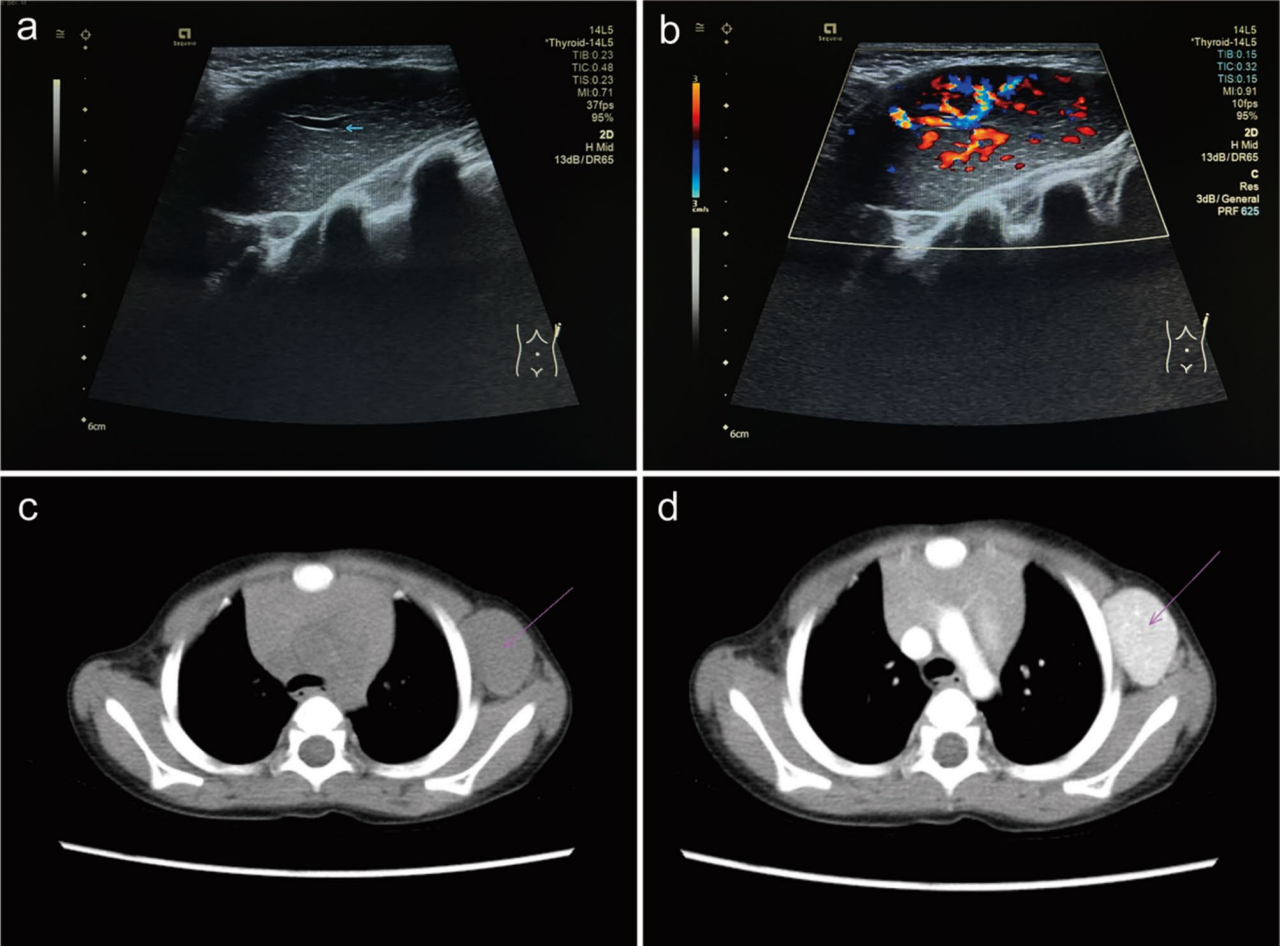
Representative imaging pictures of a left axillary mass in unicentric Castleman disease. a Ultrasonography image showed a homogeneous hypoechoic area with a regular shape and sharp border and a thick blood supply vessel passing through the mass (blue arrow); b color Doppler flow imaging detected rich blood flow signals in the mass; c general CT image showed a homogeneous mass with similar density to soft tissue (purple arrow), and the CT value was 55 Hu; d the mass was significantly and homogeneously enhanced after contrast injection, and the CT value was 160 Hu
